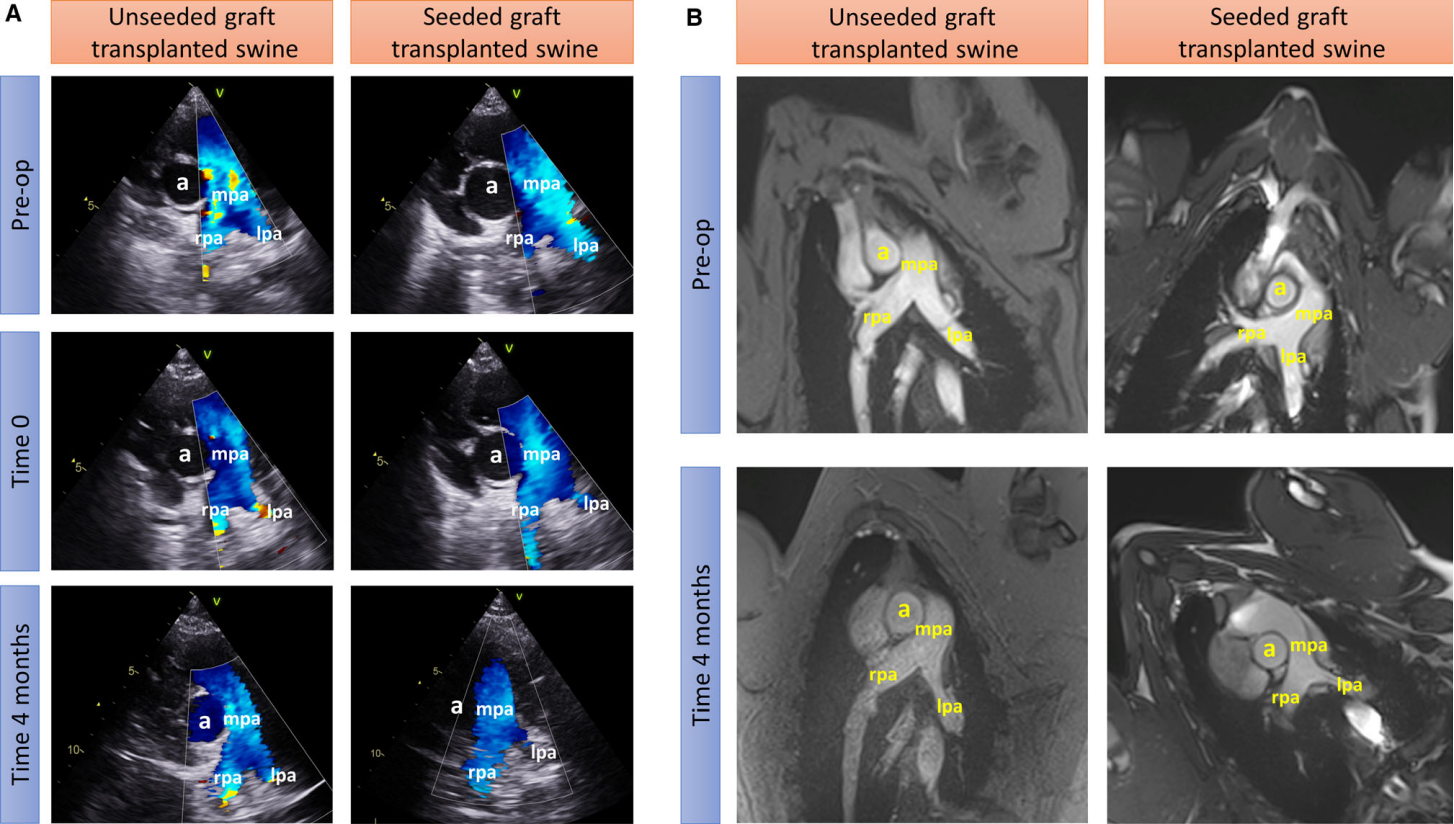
Imaging studies using Doppler echocardiography (A) and cardiac MRI (B). Vascular structures are highlighted (a) aorta, (mpa) main pulmonary artery, (rpa) right pulmonary artery, and (lpa) left pulmonary artery. Preop indicates preoperation.
